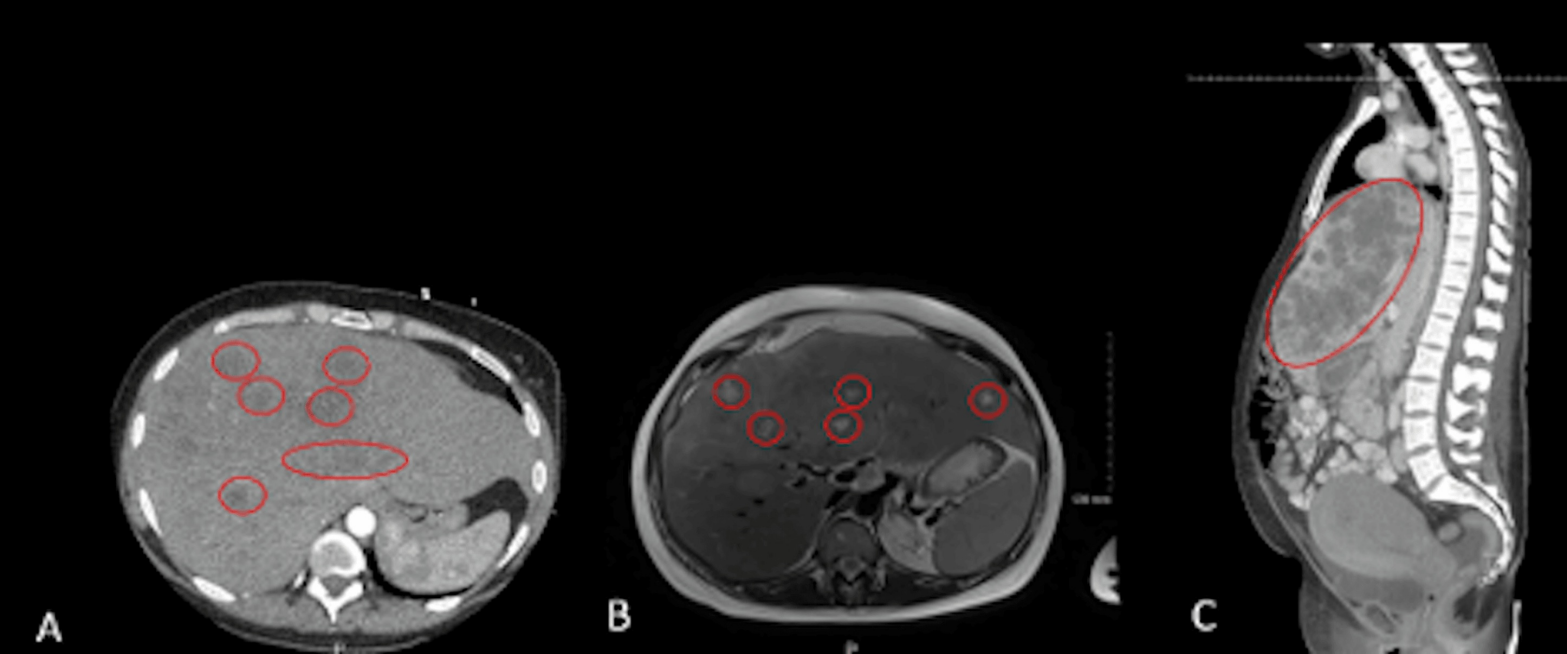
A: CT of the thorax showing multiple nonspecific hypodense liver lesions. B: Abdominal MRI and MR cholangiopancreatography showing abnormally enlarged liver with edema and inflammation as well as multiple liver lesions. C: Postpartum CT of the abdomen/pelvis showing extensive tumor involvement of the liver. CT: computed tomography, MRI: magnetic resonance imaging, MR: magnetic resonance
